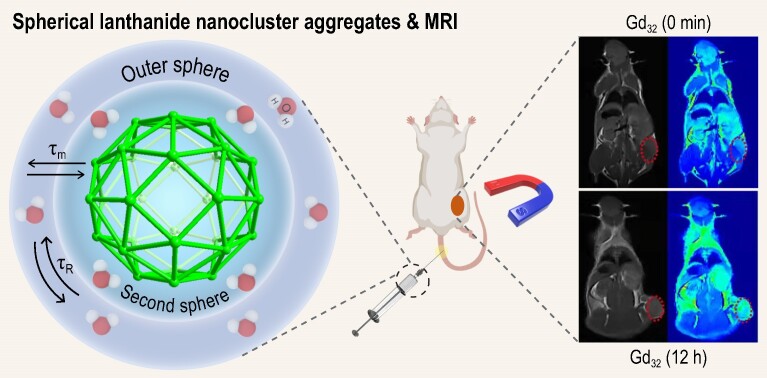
Spherical nanocluster Gd_32_ acts as a *T*_1_-weighted MRI CA for the diagnosis of tumors.
